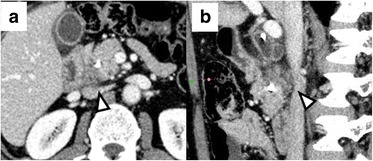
Preoperative computed tomography (CT) imaging of case 2. a An irregular tumor was identified in the pancreatic head and extended to the dorsal border of the pancreas (*arrowhead*). b Sagittal multi-planar reformation showed that the lesion adhered to and deformed the inferior vena cava (IVC)
